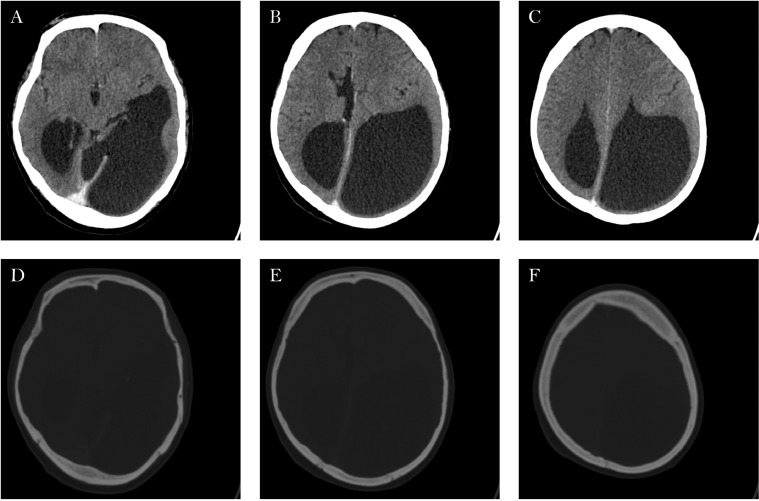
Axial CT scan reveals the enlarged ventricles (A, B, C); In the bone window, we can observe the skull's deformation (D, E, F).
